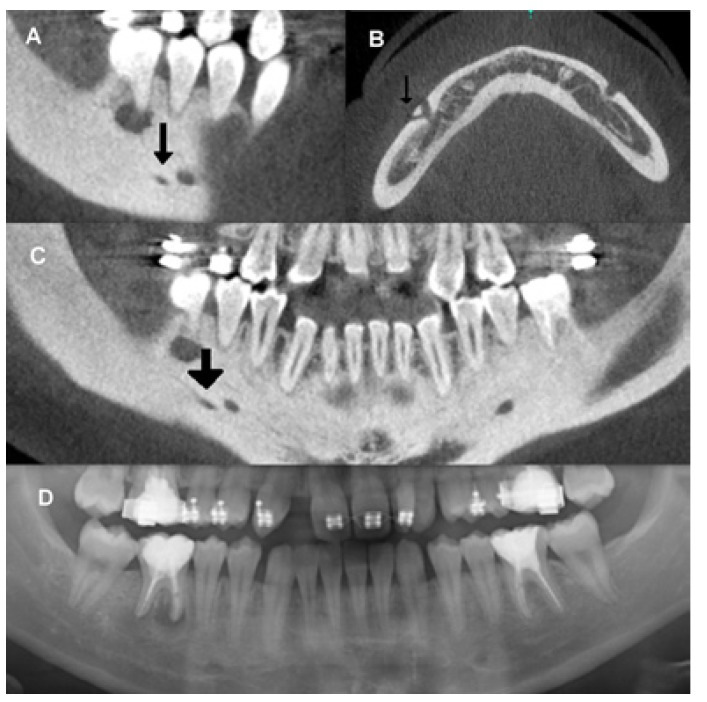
Comparison between cone beam CT images (A,B,C), and panoramic radiograph (A) of the same patient’s mandible. Sagittal (A), axial (B) and para-panoramic reconstructions revealed a posterior accessory branch of mental nerve emerging through an accessory mental foramen. Only the mental foramen is visualized with panoramic radiography (D).
